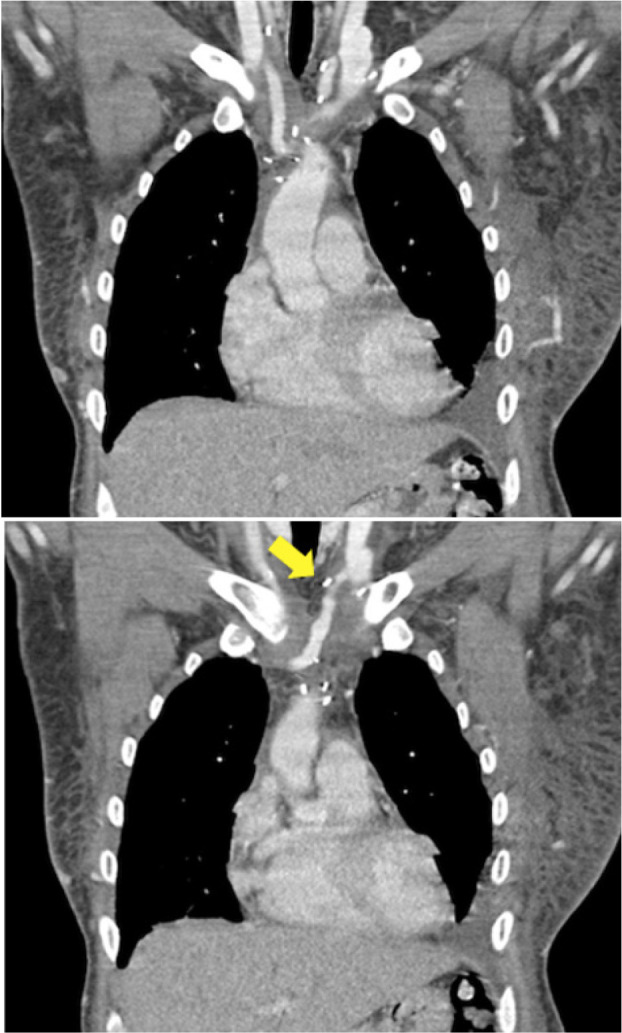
One-year follow-up venous computed tomography angiography images
